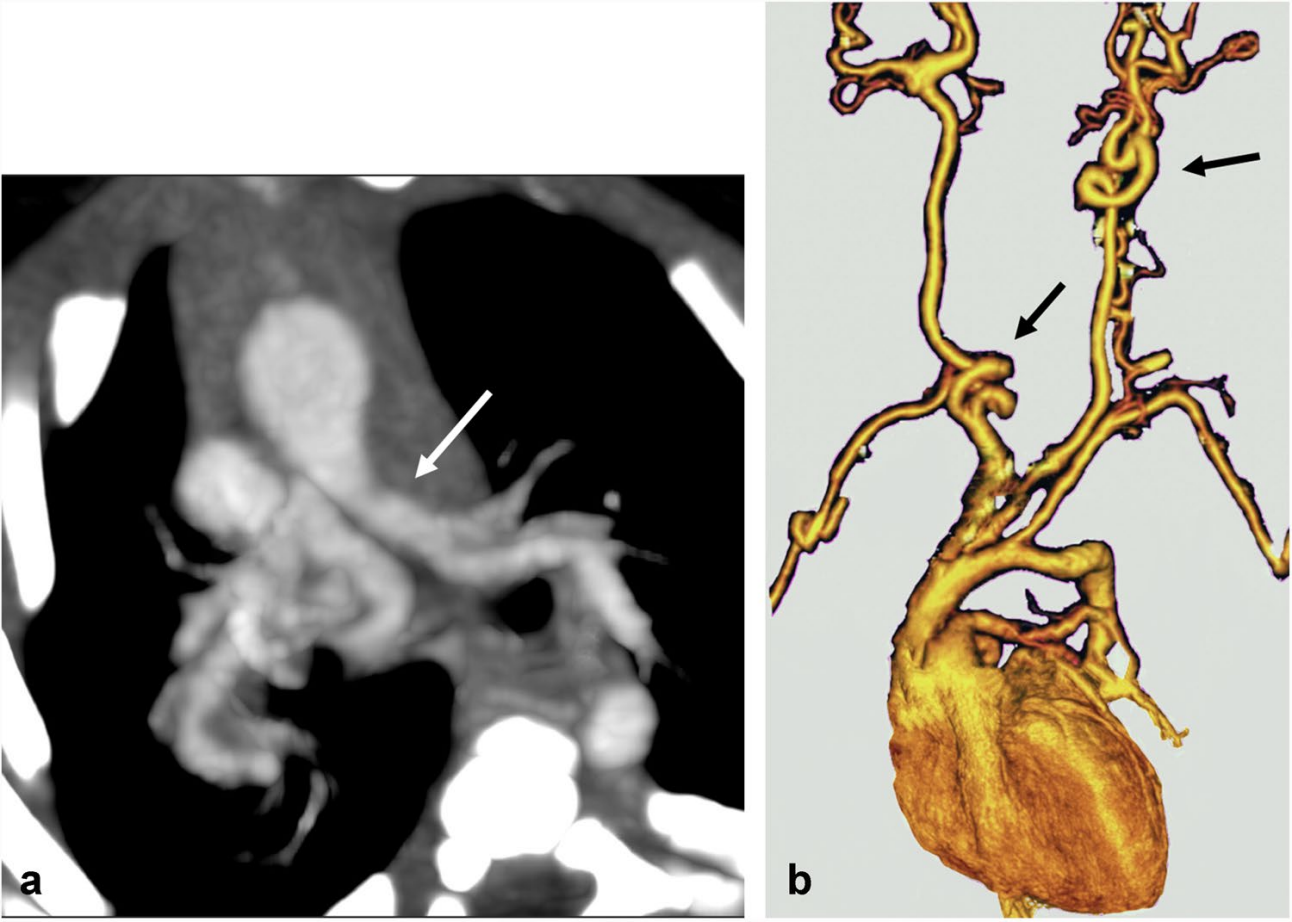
A 2-day-old girl presented with cutis laxa, frequent vomiting, and infant respiratory distress syndrome, necessitating neonatal intensive care. Echocardiography showed aortic elongation. An anteroposterior chest radiograph demonstrated an enlarged cardiomediastinal silhouette and a retrocardiac lucency due to a large diaphragmatic hernia, which was confirmed by computed tomography angiography (CTA).
